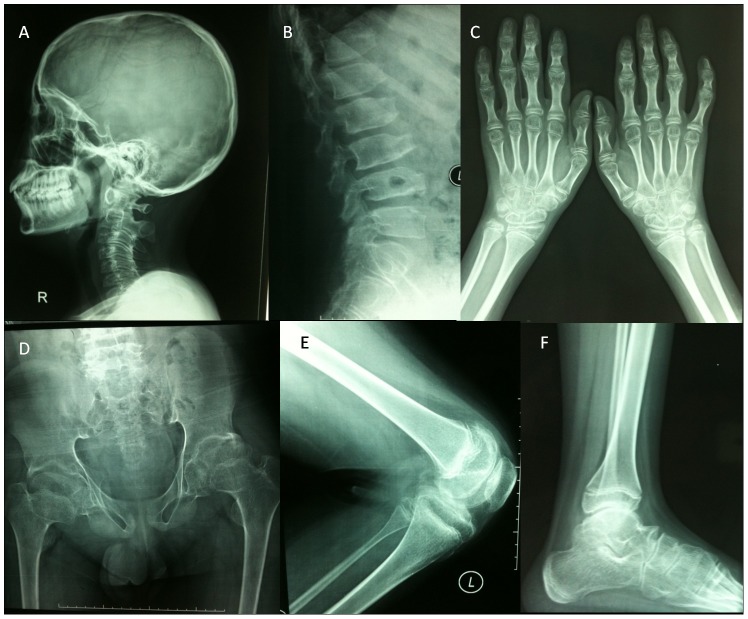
The X-ray images of patient II 1 in family 2. (A) Flattened vertebrae on the cervical spine and normal skull; (B) flattened vertebrae on the thoracic and lumbar spine with anterior beaking and an increased anteroposterior diameter of the vertebral bodies; (C) enlargement of the interphalangeal, metacarpal and metacarpophalangeal epiphyses; (D) narrow joint spaces and irregular articular surfaces of hip joints with short femoral necks; (E) slight enlargement of elbow epiphyses and metaphyses; (F) flattened talus with narrow joint spaces.
